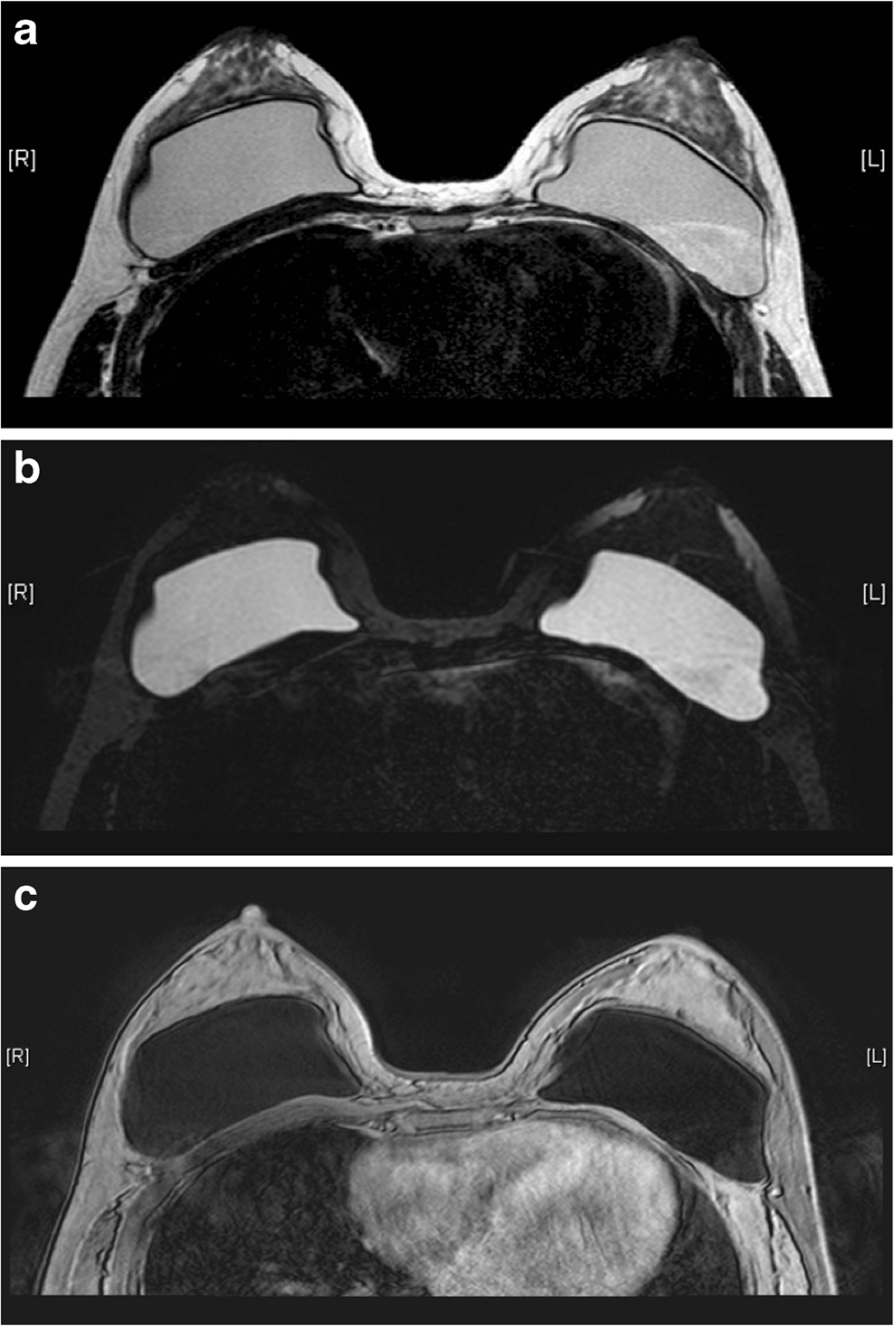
Silicone gel-filled implants. T2-weighted (a), silicone-only (b), and silicone-saturated (c) axial MR images show the specific signal intensities of silicone, thus rendering the differentiation between silicone gel-filled and saline-filled implants possible
